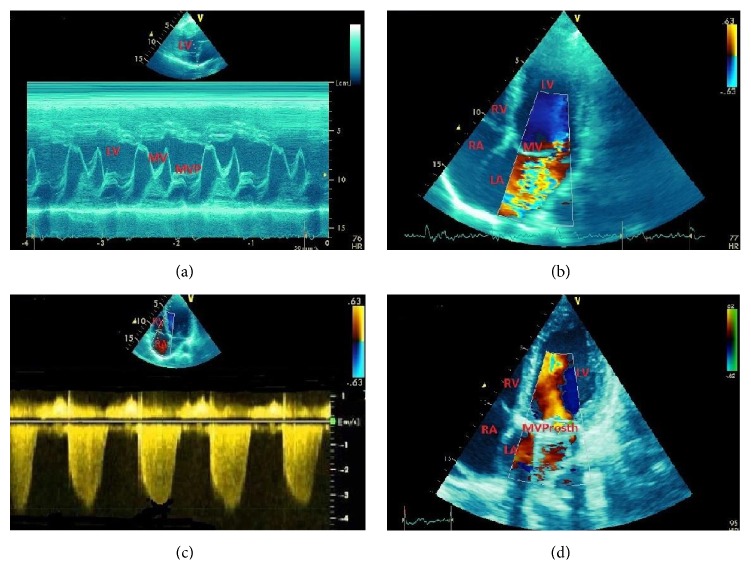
A 38-year-old male patient with congenital mitral valve prolapse (MVP), mitral regurgitation (MR), and progressive dyspnea. Patient had mitral valve surgery. (a) MVP by M-mode echocardiography. (b) Color Doppler in apical 4-chamber view showing severe MR. (c) Doppler-estimated systolic pulmonary artery pressure (58 mmHg). (d) MR reduction after mitral valve surgery (MV mechanical prosthesis). LA = left atrium; LV = left ventricle; MV = mitral valve; RA = right atrium; RV = right ventricle.
